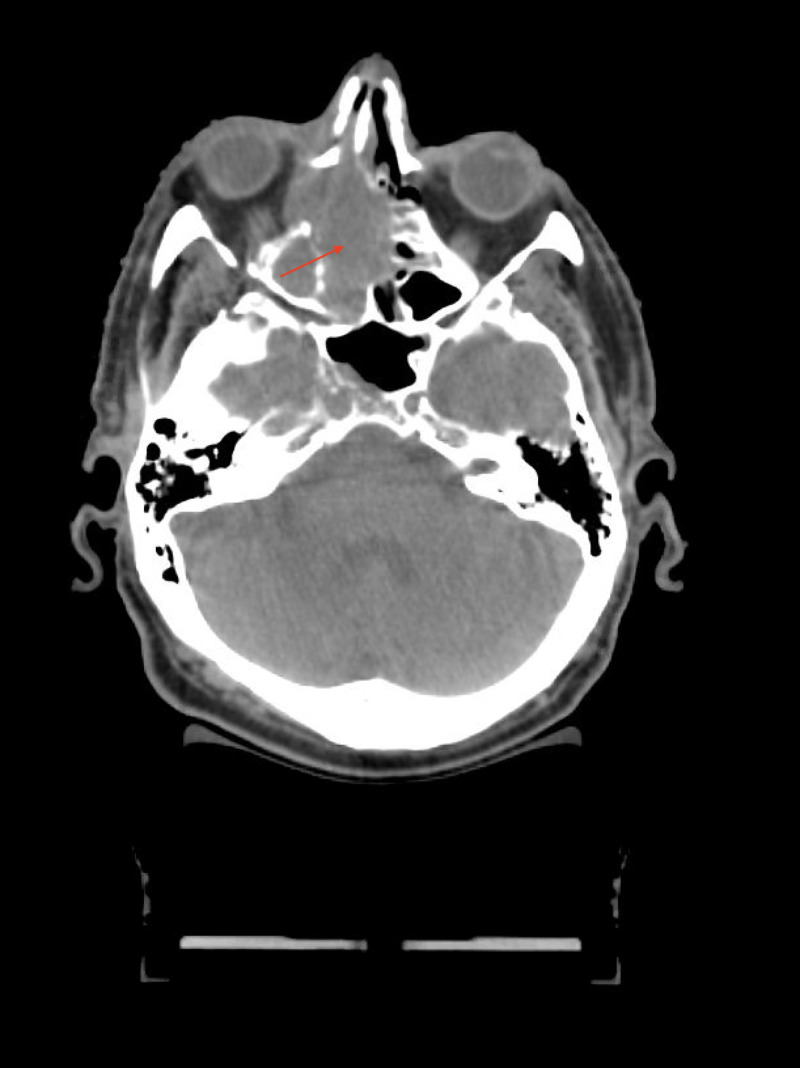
CT head noncontrast showing infiltrative mass in the right nasal cavity eroding through the right lamina papyracea into the right orbit. Red arrow: mass.
